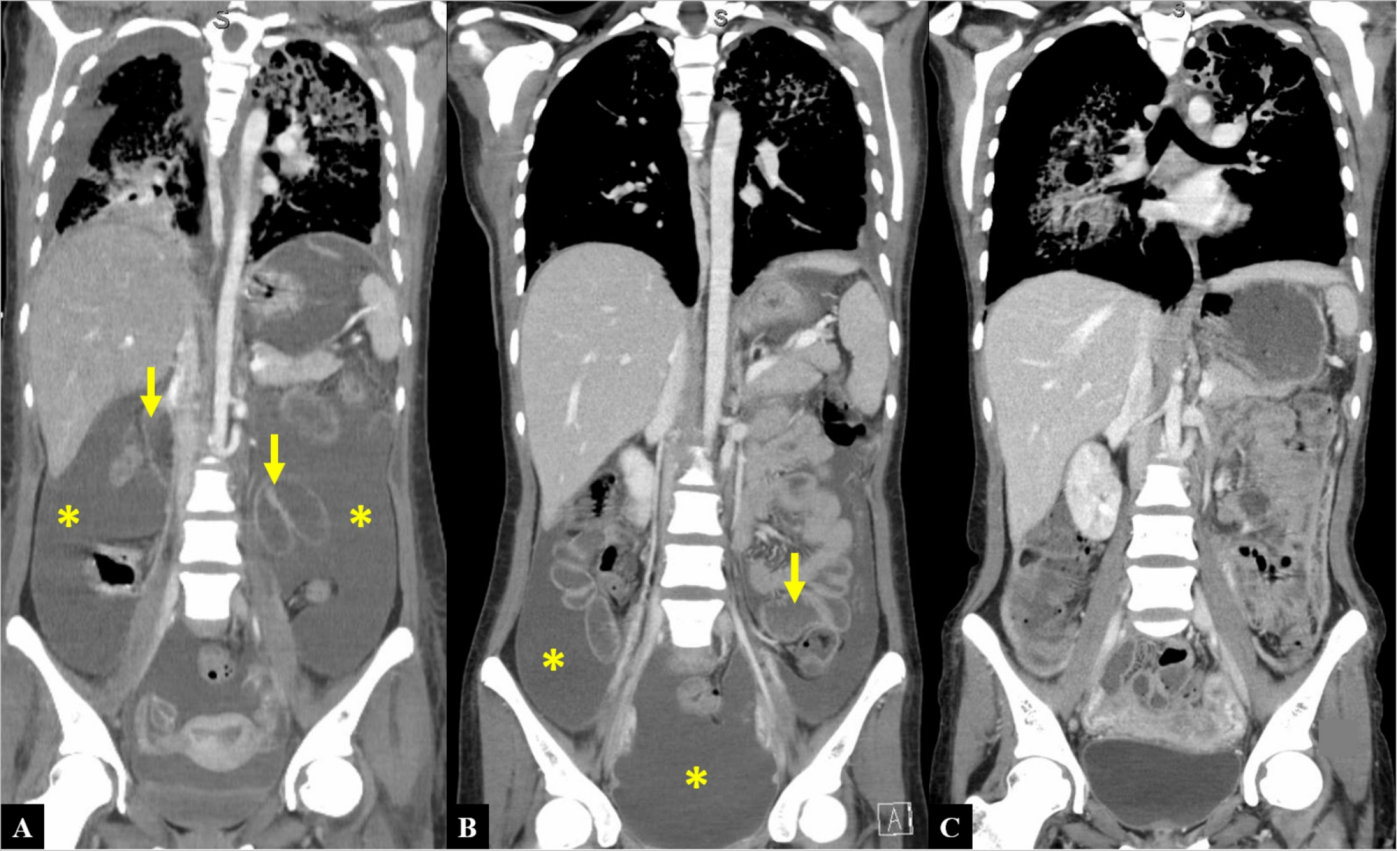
CT of the chest, abdomen, and pelvis at different clinical time points A) Depicts “...large amount of abdominal ascites present with areas of omental thickening... Mild dilatation of small bowel loops with fluid and gas…”. Ascites identified by asterisks. Bowel look dilations identified by arrows. B) Two weeks from initial CT. C) Eight weeks from initial CT CT: computed tomography
